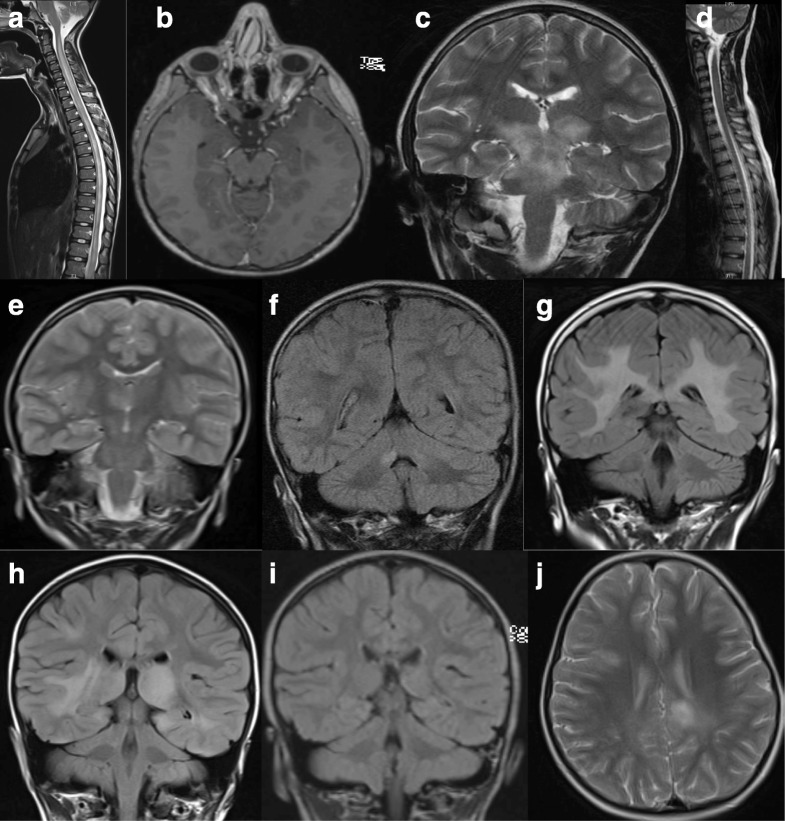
MRI findings in children with MOG-Ab-associated disease. A 10-year-old boy presented with encephalopathy, limb weakness (but able to walk), bilateral optic neuritis with only light perception, and urinary incontinence. MRI revealed longitudinally extensive transverse myelitis (a) and bilateral optic neuritis with enhancement (b). He relapsed 4 months later with headaches, fever, and encephalopathy. MRI revealed confluent lesions involving the pons, midbrain, and the superior cerebellar peduncles (c) as well as the left frontal opercular cortex. The previous spinal lesion has significantly improved (d). A 4-year-old girl presented with encephalopathy, seizures, and lethargy progressed to weakness of her right arm and bilateral 6th nerve palsies. MRI demonstrated a focus of signal change in the right thalamus (e). She relapsed 4 months later with brainstem cerebellar syndrome and lesions in both middle cerebellar peduncles (f) as well as a new lesion in the left side of the midbrain (not shown). At 4 years of age, she presented with bilateral optic neuritis. MRI showed diffuse bilateral white matter signal abnormality involving the cortical and subcortical regions extending inferiorly along the cerebral peduncles, pons, and right middle cerebellar peduncle, as well as swelling and signal abnormality of the corpus callosum (g). She had a further episode of bilateral optic neuritis 1 year later. A 4-year-old girl presented with encephalopathy, headache, and lethargy and MRI findings revealed diffuse bilateral and asymmetric signal abnormalities of the basal ganglia and thalamus with similar non-enhancing disease of the cortex, subcortical and deep white matter of both hemispheres (h). Repeat imaging 3 months later demonstrated near complete resolution of all lesions, concurrent with clinical recovery. She relapsed 15 months later with new onset headaches, encephalopathy characterized by behavioral change with hallucinations and confusion, and MRI evidence of new cortical and juxtacortical T2 lesions (j).
